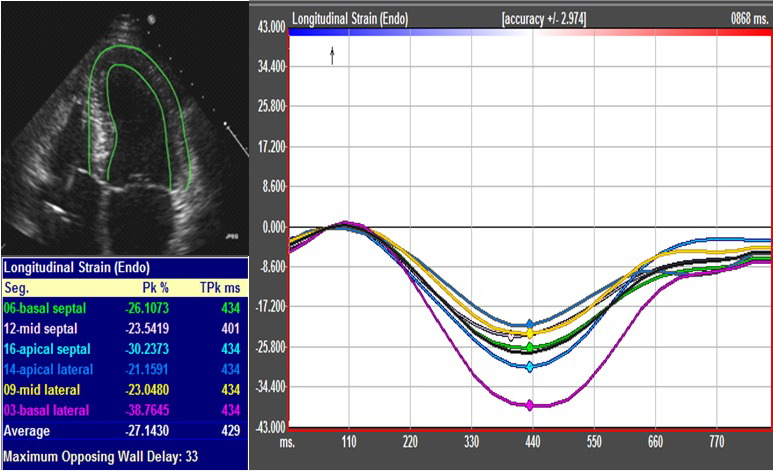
Longitudinal strain curves from left ventricle (LV) apical four‐chamber view in a normal subject using speckle tracking echocardiography. Endocardial borders were traced, and myocardial walls were tracked. The interactive software then automatically tracked myocardial motion during the entire LV mechanical cycle and divided each image into six segments. LV was divided into 18 segments with six segments per view, and global longitudinal strain (GLS) was averaged over all the trackable segments
